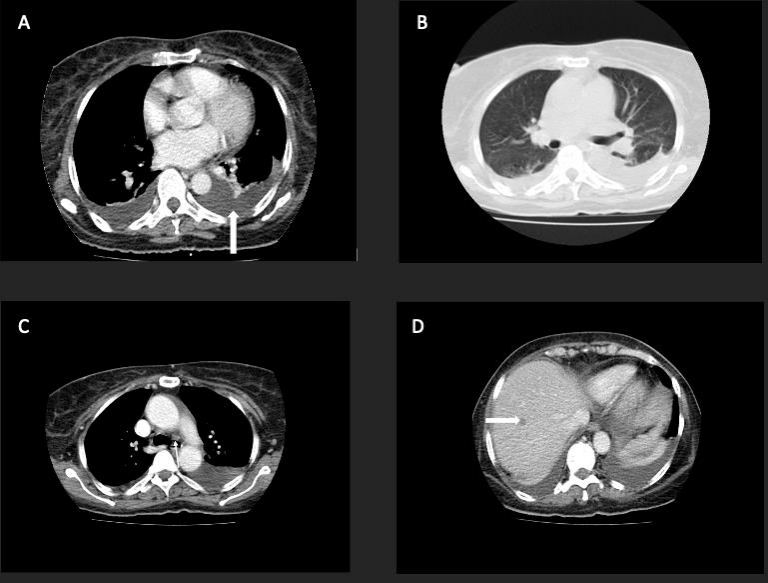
Contrast-enhanced axial computer tomography (CT) scan of the chest of the index patient. A: The mediastinal window shows moderate bilateral pleural effusion (left pleural effusion white arrow). B: No lung nodules are seen in the lung window. C: A small prominent pre-tracheal lymph node ∼ 6mm short axis dimension (arrowed), (mediastinal window), and D: Axial projection shows a small, well-defined non-enhancing hypodense lesion in the liver measures 9.3mm (arrowed)
